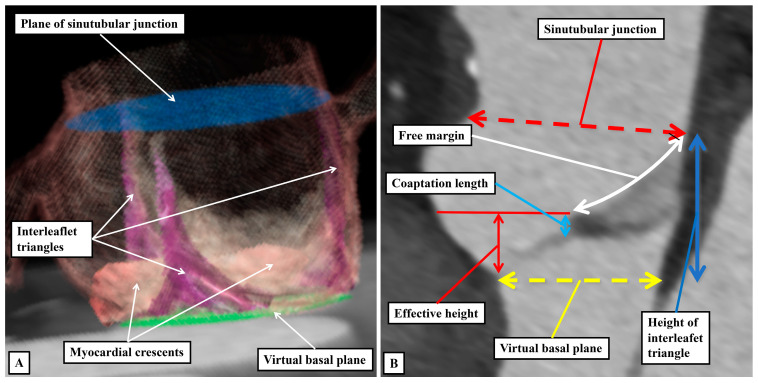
(A) shows a three-dimensional reconstruction of the aortic root using a computed tomographic dataset of a normal individual. The interleaflet triangles have been segmented, along with the myocardial crescents at the bases of the valvar sinuses which give rise to the coronary arteries. The planes of the sinutubular junction and the virtual basal ring have also been segmented. (B) shows a two-dimensional section of a different computed tomographic dataset taken through the non-coronary sinus. It shows the detailed metrics that can now be taken for the purposes of repair of diseased aortic roots.
